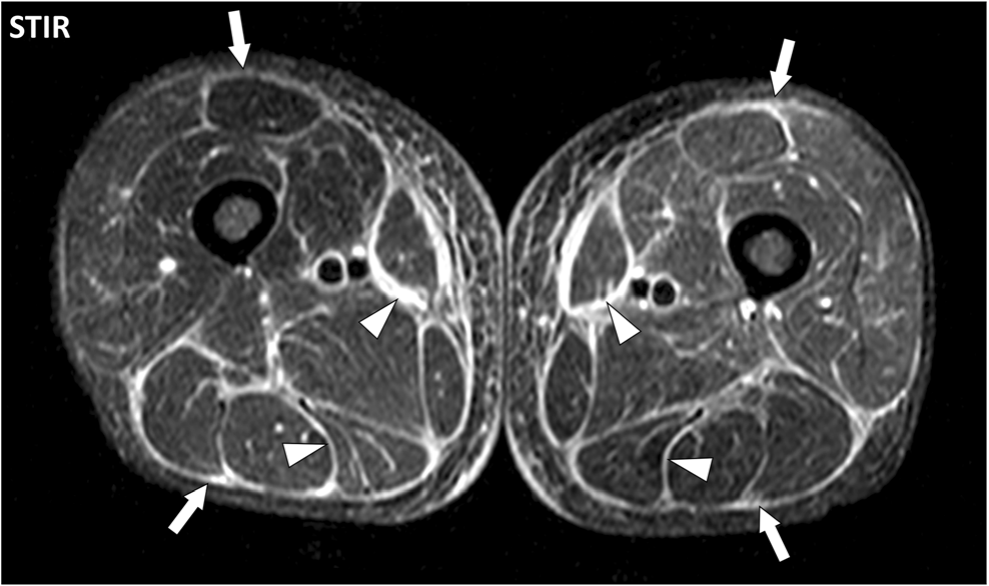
Axial STIR image of the thighs of a 50-year-old male with eosinophilic fasciitis. MRI demonstrates bilateral symmetrical diffuse involvement of the deep peripheral fasciae (arrows) and deep intermuscular fasciae (arrowheads), which are thickened with high signal intensity. The fascia superficialis is barely involved and the signal of the muscles is normal
